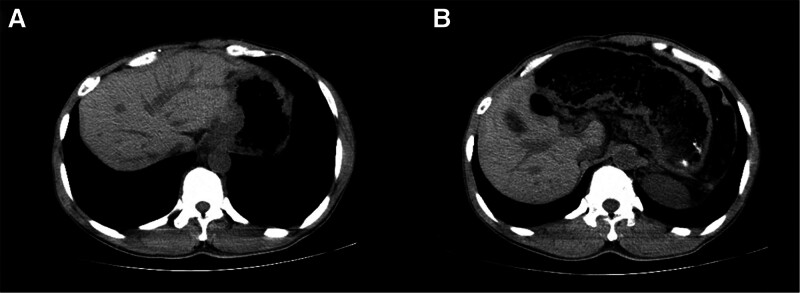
Abdominal CT revealed cirrhosis, liver lobar imbalance, and widened portal vein in Case 2. CT = computed tomography.
